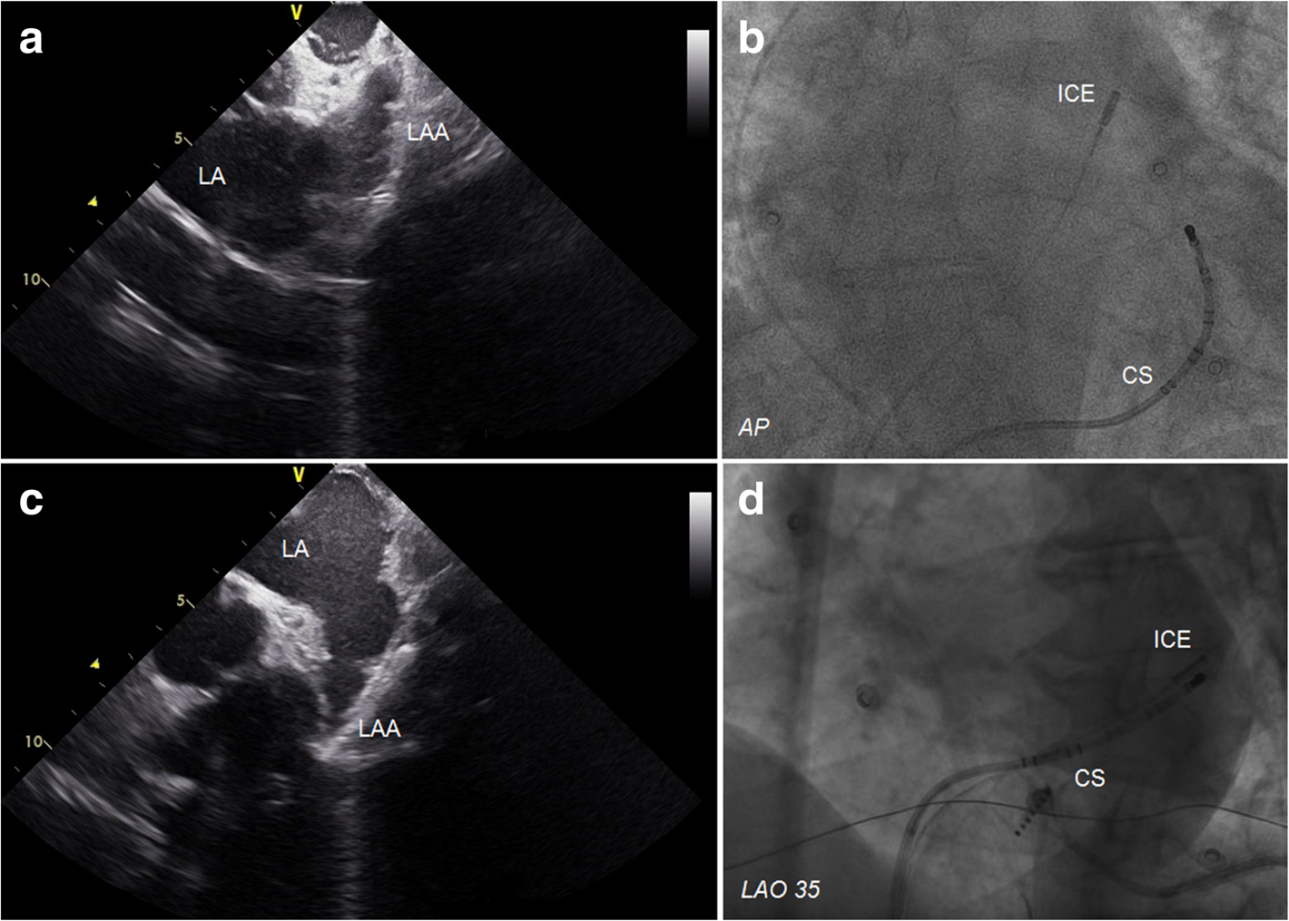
The upper row is demonstrating an example of the intracardiac echocardiographic image of the left atrial appendage from the pulmonary artery (a), and a fluoroscopic view of the intracardiac echocardiography probe in the pulmonary artery (b). The lower row is demonstrating an example of the intracardiac echocardiographic image of the left atrial appendage from the coronary sinus (c), and a fluoroscopic view of the intracardiac echocardiography probe in the coronary sinus (d)
